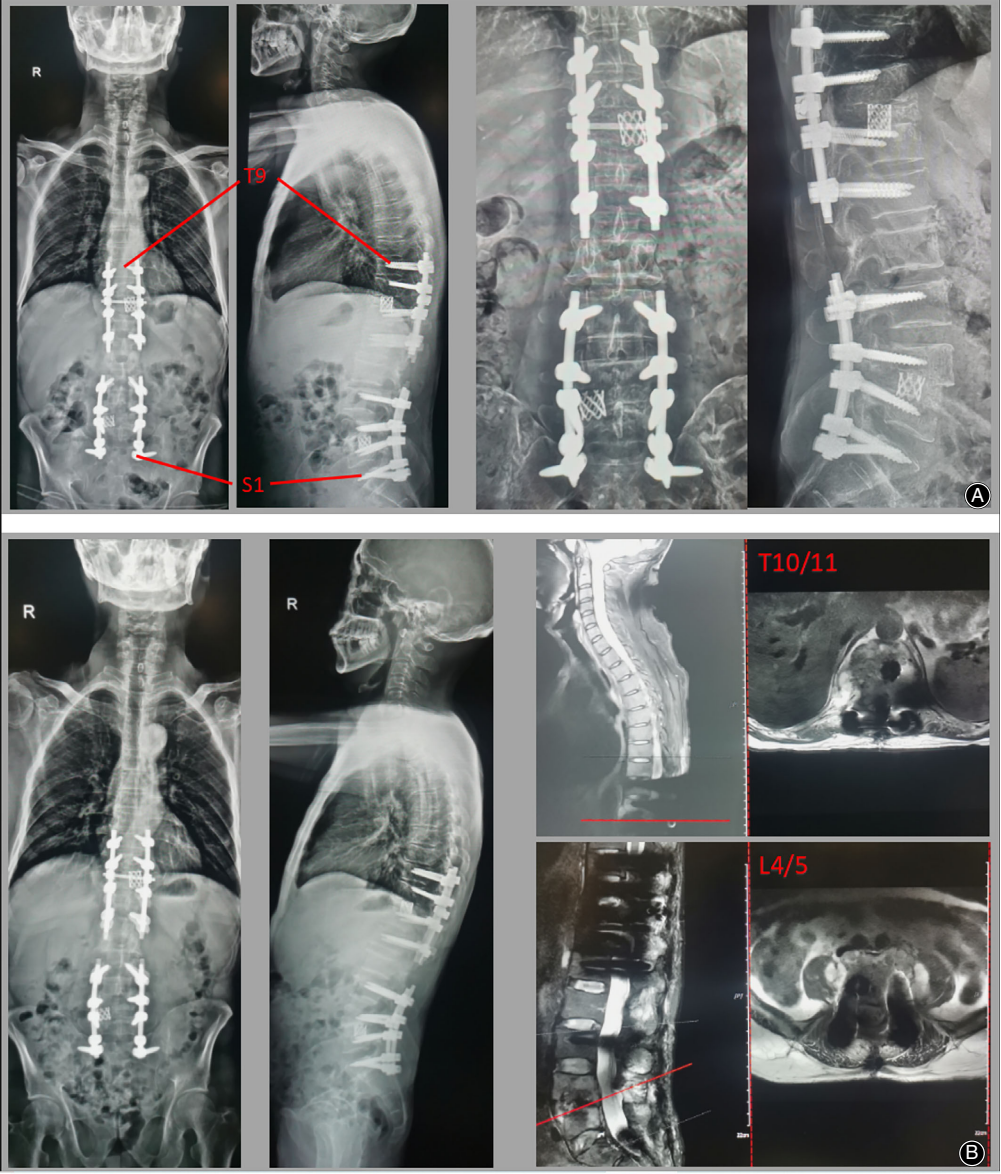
(A) The postoperative X‐ray showed the kyphosis was significantly corrected. (B) X‐ray 4 months after the operation indicated the titanium cage was fixed in place and MRI showed the spinal canal was thoroughly decompressed.
